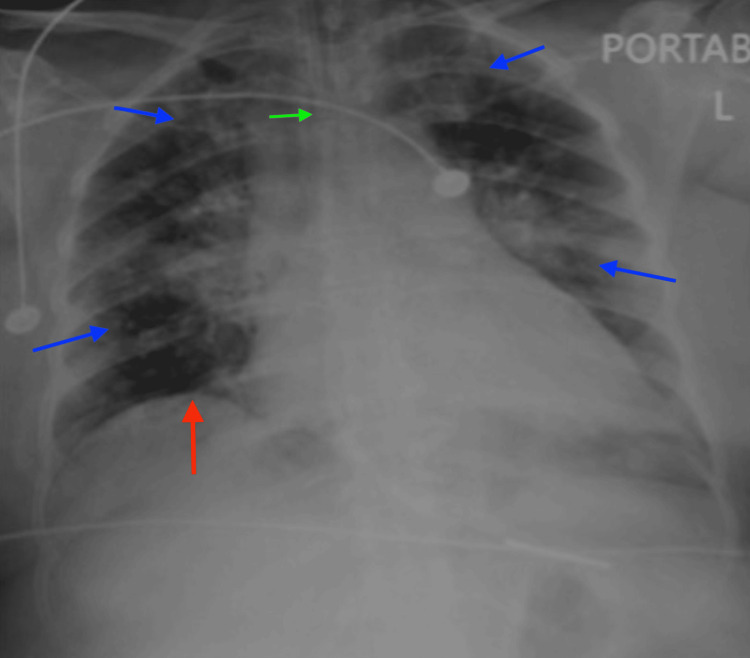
Post-Intubation Chest Radiograph Post-intubation chest radiograph demonstrating appropriate endotracheal tube placement and progression of bilateral pulmonary edema. The red arrow indicates persistent elevation of the right hemidiaphragm. The blue arrows indicate new bilateral interstitial and alveolar opacities consistent with pulmonary edema. The green arrow indicates the endotracheal tube in appropriate position.
